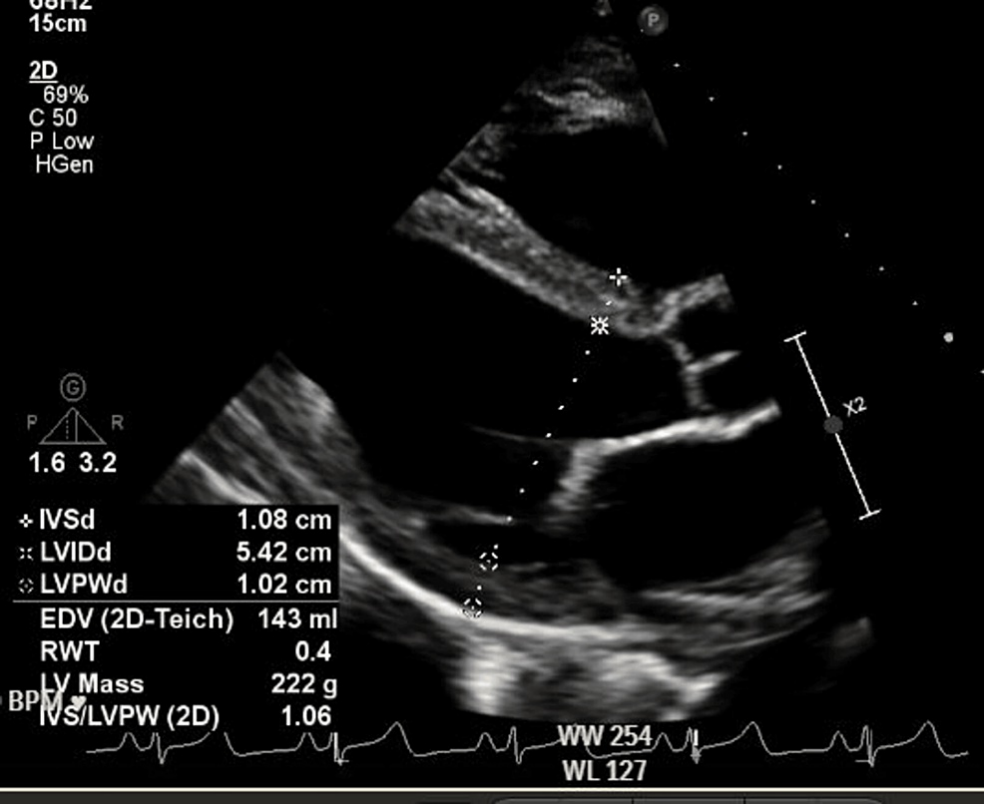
Further cardiac measurements in the parasternal long-axis view
